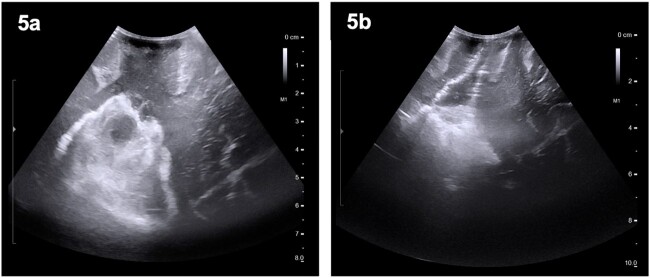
Preoperative (5a) ultrasound images depicting a heterogenous mass with hyperechoic and hypoechoic regions. Post-operatively (5b), a hyperechoic region is noted; however, no distinct lesion is identified.
